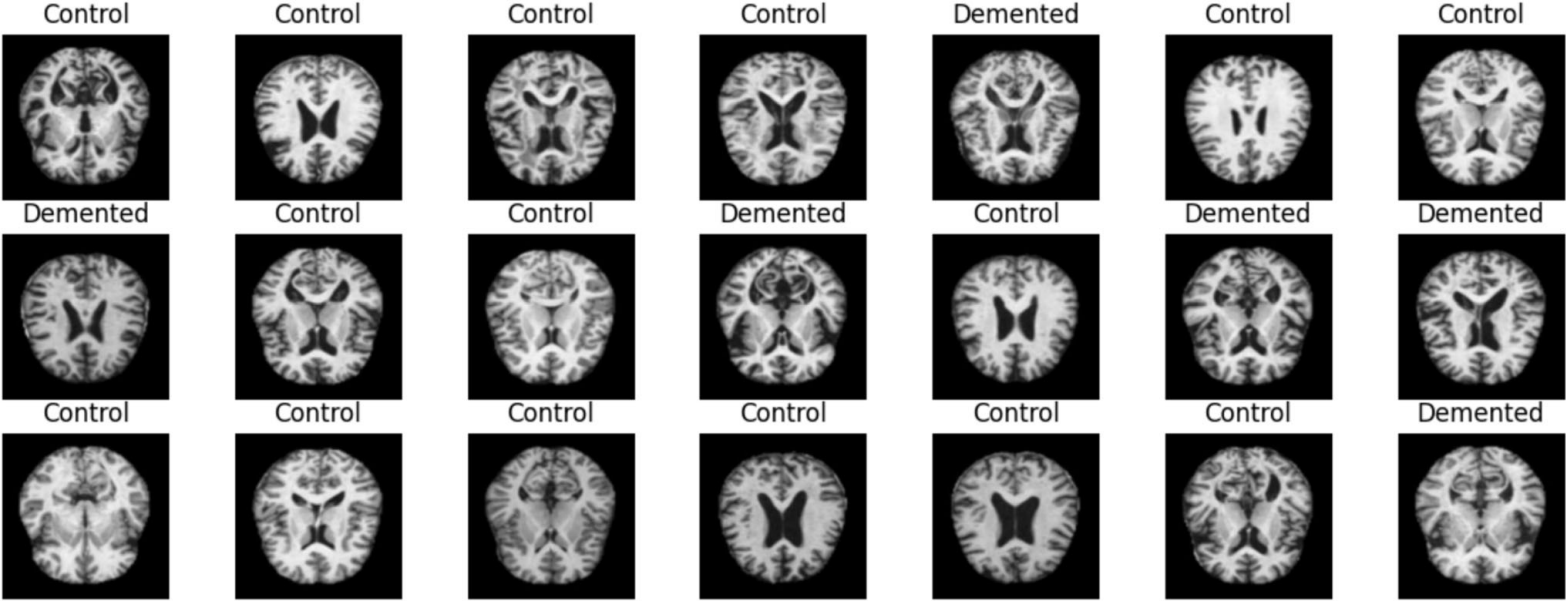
Example MRI images labeled with and without dementia in Dataset 5
